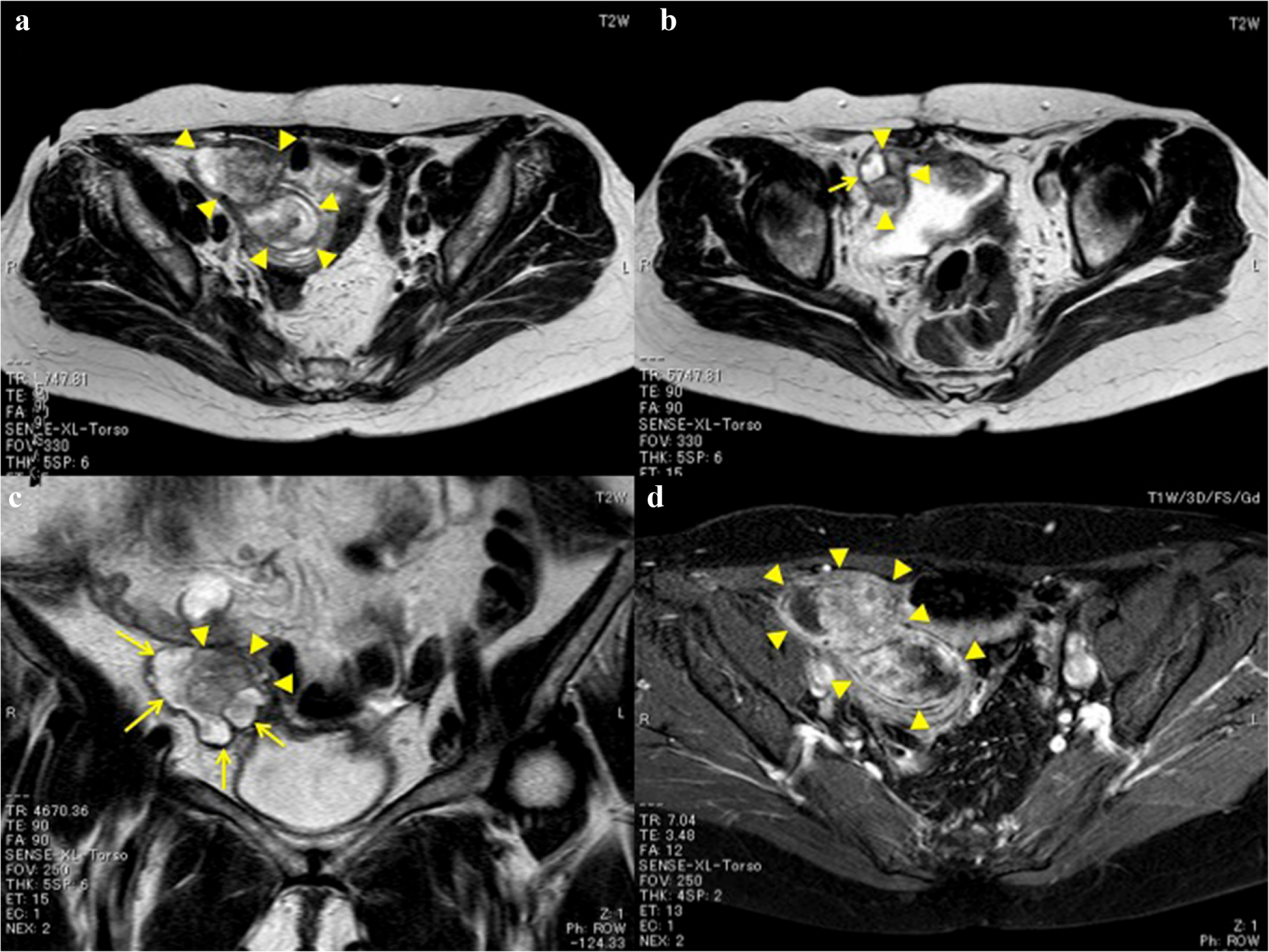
MR imaging. a T2-weighted imaging. A lobulated tumor was observed ventral to the right common iliac artery. The tumor had heterogeneous intensity. b, c T2-weighted imaging. Some high-intensity cystic lesions (*arrow*) were detected around the tumor. d T1-weighted imaging. The mass had heterogeneous enhancement (*arrowheads* indicate the outline of the tumor)
